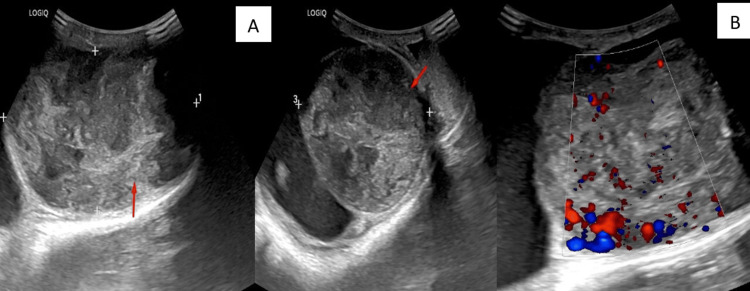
Two-dimensional B-mode ultrasound image and colour Doppler image Bulky testis entirely replaced by heteroechoic mass lesion (red arrows in A) with increased vascularity (B).
